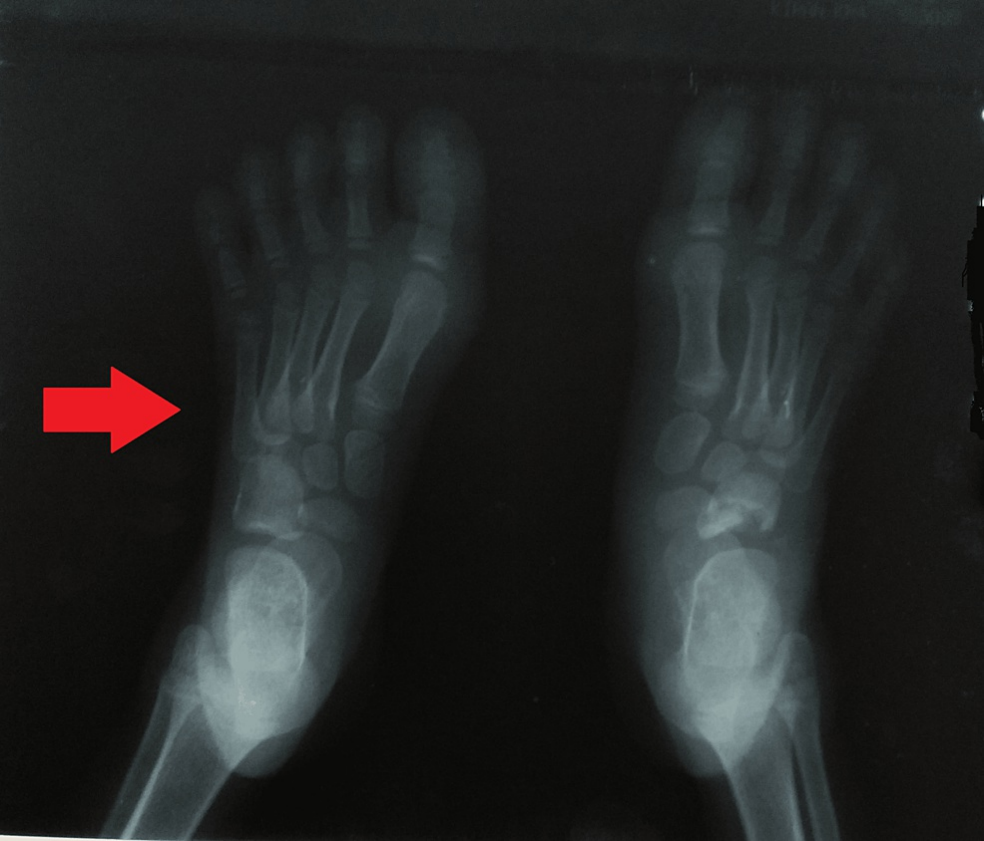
Radiograph of the feet revealed bilateral hallux valgus deformity.
